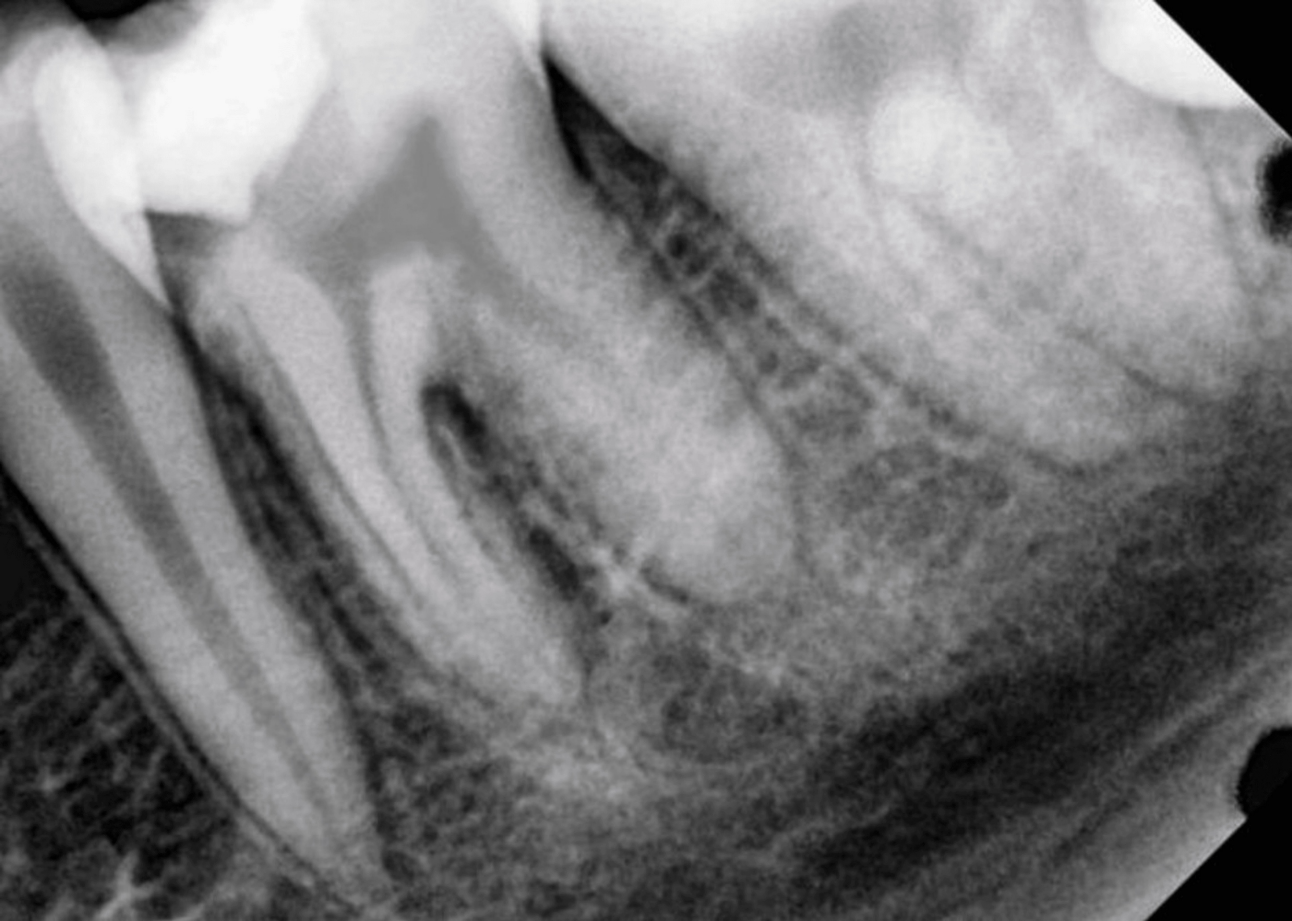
Periapical radiograph of tooth #36 with a temporary restoration
